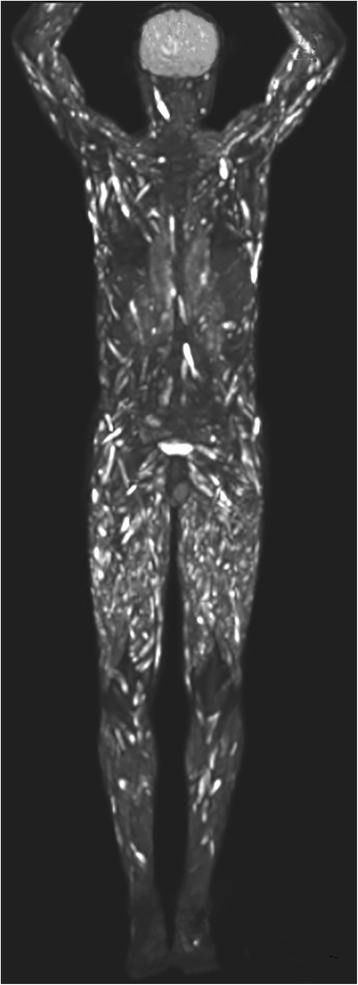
Coronal reconstruction of a positron emission tomography/computed tomography examination shows increased fludeoxyglucose uptake with diffuse involvement of the muscles of the extremities and the trunk
